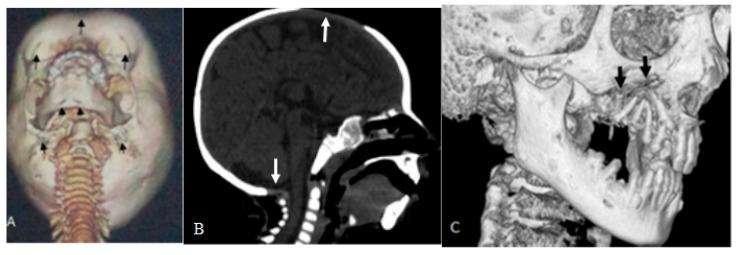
A 3D reconstruction of the craniofacial bones in extension (dynamic) in a 13-year-old girl with GS showed mid-line defective ossification of the metopic suture, causing bifid/bossing of the frontal area, bilateral bossing of the suprazygomatic arch, defective dentition of the lower jaw, several bony pits over the mandible, and the lambdoid area-arrow heads (A). A 3D sagittal reformatted CT scan of the skull of a 20-month-old girl with GS showed defective ossification of the cranial vault (arrow) and delayed closure of the posterior fontanelle (arrow) (B). A cranio-facial reconstruction CT scan of a 16-year-old girl with GS showed marked cystic cavitation of the temporal bone associated with excessive lytic changes of the upper jaw (arrows) and the mastoid, causing tremendous frailty of the mastoid bone. Note the severe dental dysplasia and oligodontia (C).
